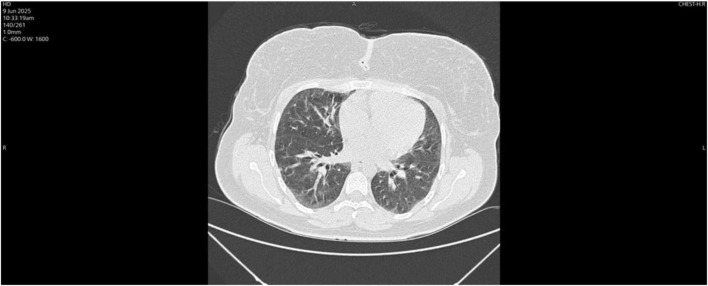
High-resolution chest CT demonstrating bilateral lower-lobe-predominant centrilobular and subpleural ground-glass opacities consistent with inflammatory interstitial lung disease.
